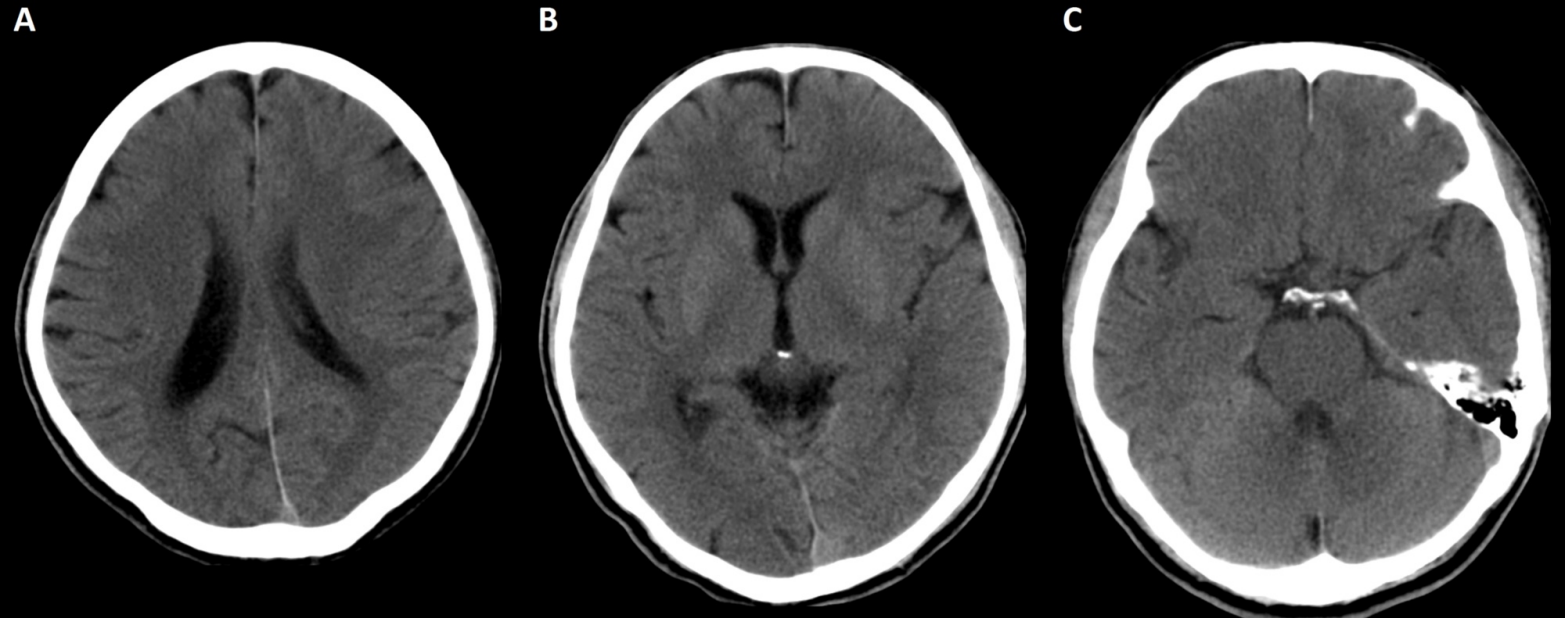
A three-month follow-up CT of the brain. There is no evidence of structural damage after three months despite the focal dystonia and mild cognitive impairment. (A) The body of the lateral ventricles cut with no evidence of chronic structural damages on the frontal, parietal, and occipital lobes. (B) The basal ganglia (the most common site for structural brain insult after cyanide toxicity) are not affected and the patient did not show any features of extrapyramidal signs. (C) No evidence of chronic ischemic changes was noted on temporal and cerebellar areas.
